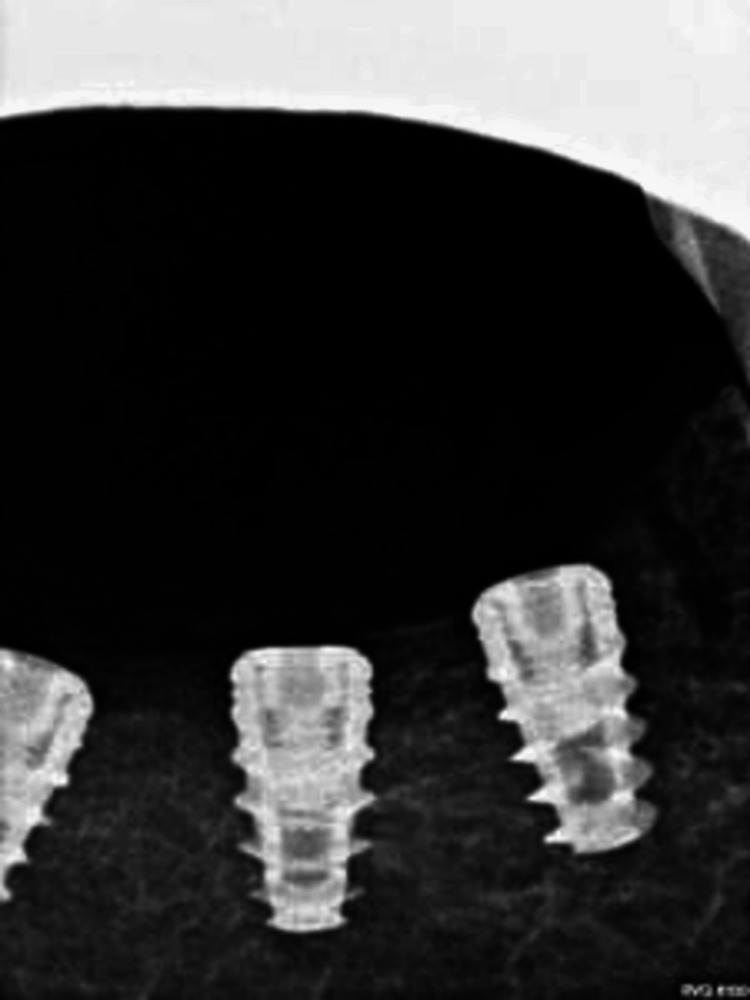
Intraoral periapical radiography of the mandibular region with implants
